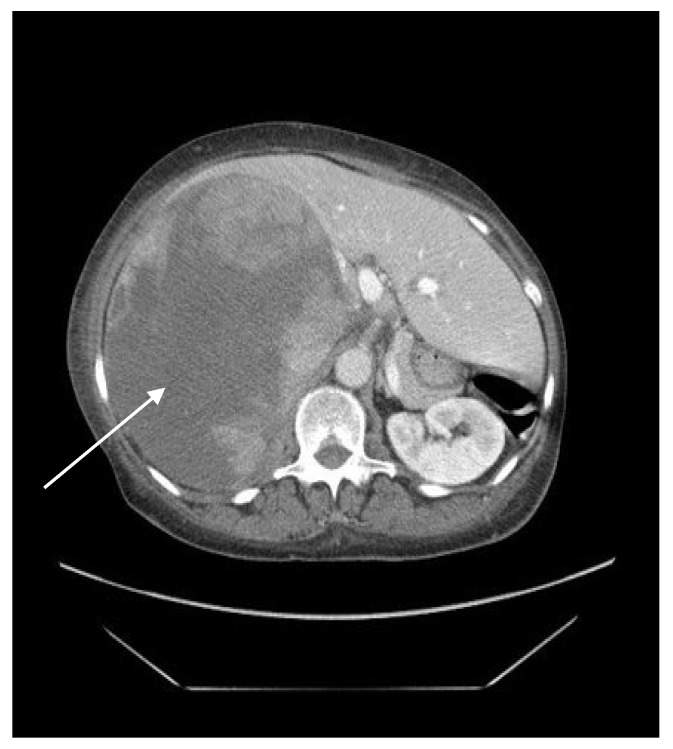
Contrast-enhanced CT scan of the abdomen and pelvis, axial view. Large heterogeneous mass visualized (arrow).
